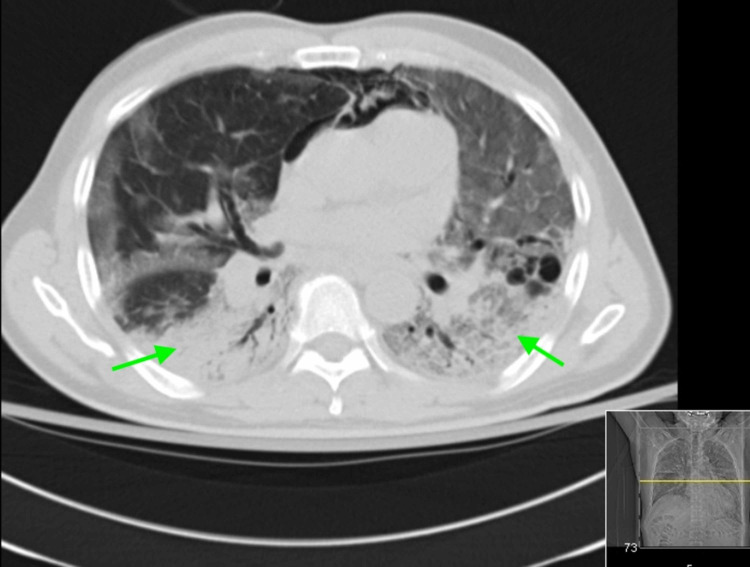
CT of the chest - image 2 The image shows ground-glass airspace opacities bilaterally (arrows) CT: computed tomography
